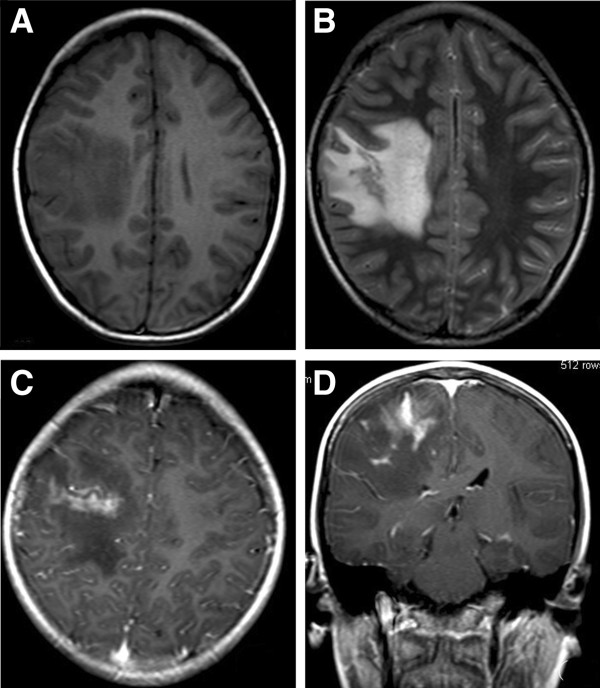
MR images for the case 2. A. The T1W image shows there is an irregular large patchy area of hypointensity in the right parietal lobe. B. The T2W image shows there is a serpiginous tubular hyperintense lesion (Black arrow) with a perilesional large patchy irregular edema (White arrow) in the right parietal lobe. The edema shows low signal on the T1W image and high signal on the T2W image. C and D. The enhanced T1W images show a serpiginous tubular enhancement for the lesion.
